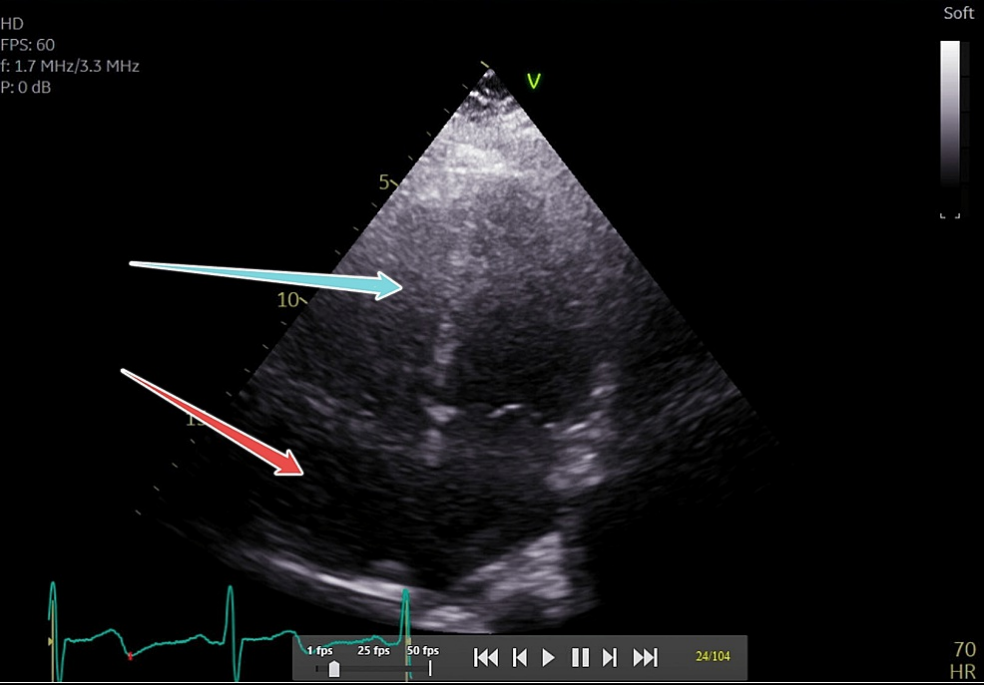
Transthoracic echocardiogram showing mild-left atrial dilation - the red arrow pointing to the right atrium and the blue arrow pointing to the right ventricle for reference
